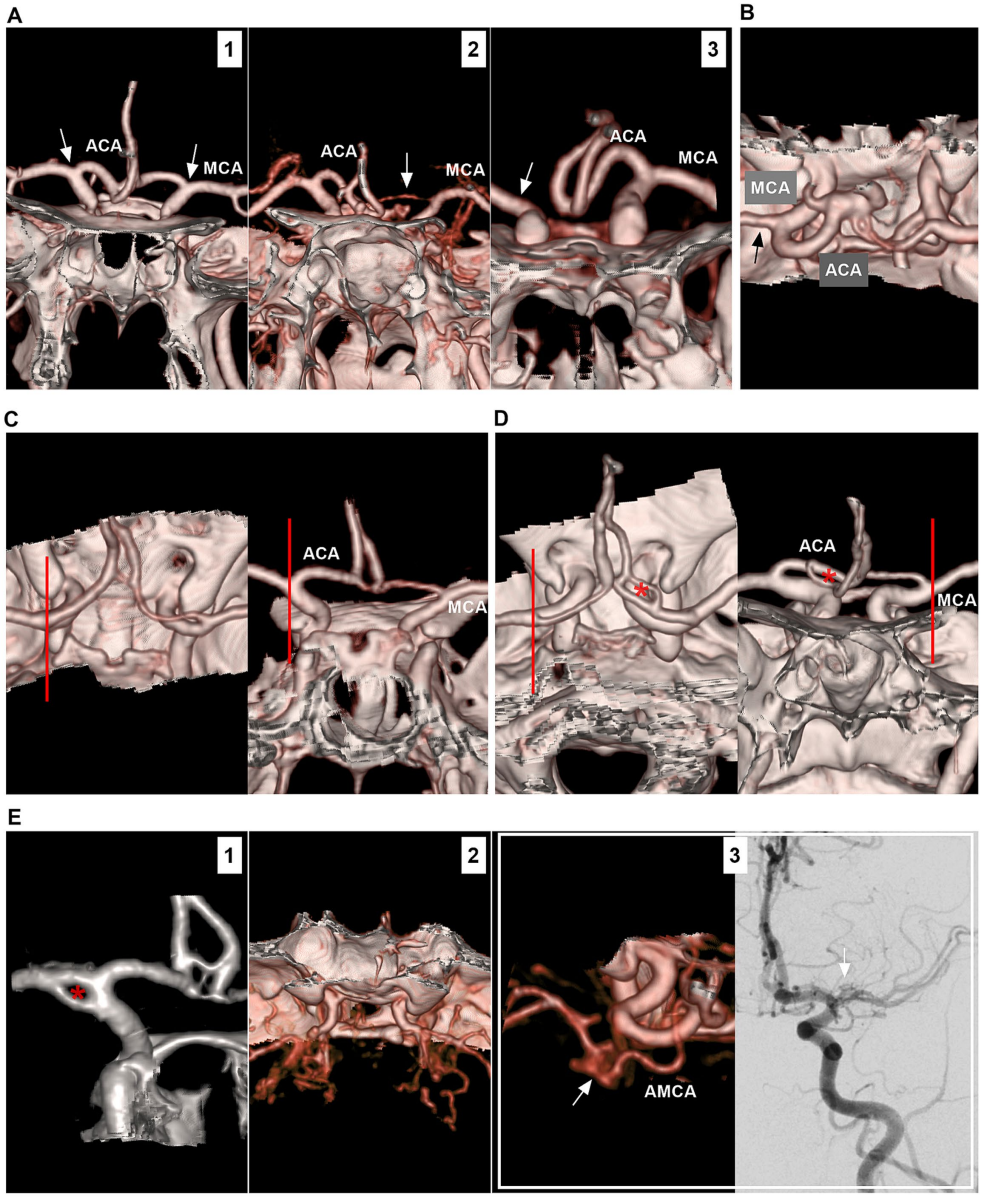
Anatomy and anomaly of the ICA bifurcation region. (A) Number 1 panel: CTA showing symmetrical ICA bifurcations (arrows), the ACA was well-developed. Number 2 panel: CTA showing asymmetrical ICA bifurcations (arrows), and the unilateral ACA (arrow) was hyperplastic. Number 3 panel: CTA showing that unilateral ACA (arrow) was absent, and there was no true ICA bifurcation (arrow). (B) CTA showing that, in the ICA bifurcation region, the ACA was thicker than the MCA (arrow). (C) In the left and right panels (different views), CTA showing the ICA bifurcation located at the level of anterior clinoid process (lines). (D) In the left and right panels (different views), CTA showing the ICA bifurcation located outside anterior clinoid process (lines); the fenestration (asterisks) on the ACA can be seen. (E) Number 1 panel: CTA showing a fenestration (asterisk) at the ICA bifurcation. Number 2 panel: CTA showing moyamoya disease involving bilateral ICA bifurcations. Number 3 panel: CTA (left) showing that the twig-like MCA (arrow); the AMCA supplied the twig-like MCA; DSA (right) showing the twig-like MCA (arrow). ACA, anterior cerebral artery; AMCA, accessory middle cerebral artery; CTA, computed tomography angiography; DSA, digital subtraction angiography; ICA, internal carotid artery; MCA, middle cerebral artery.
